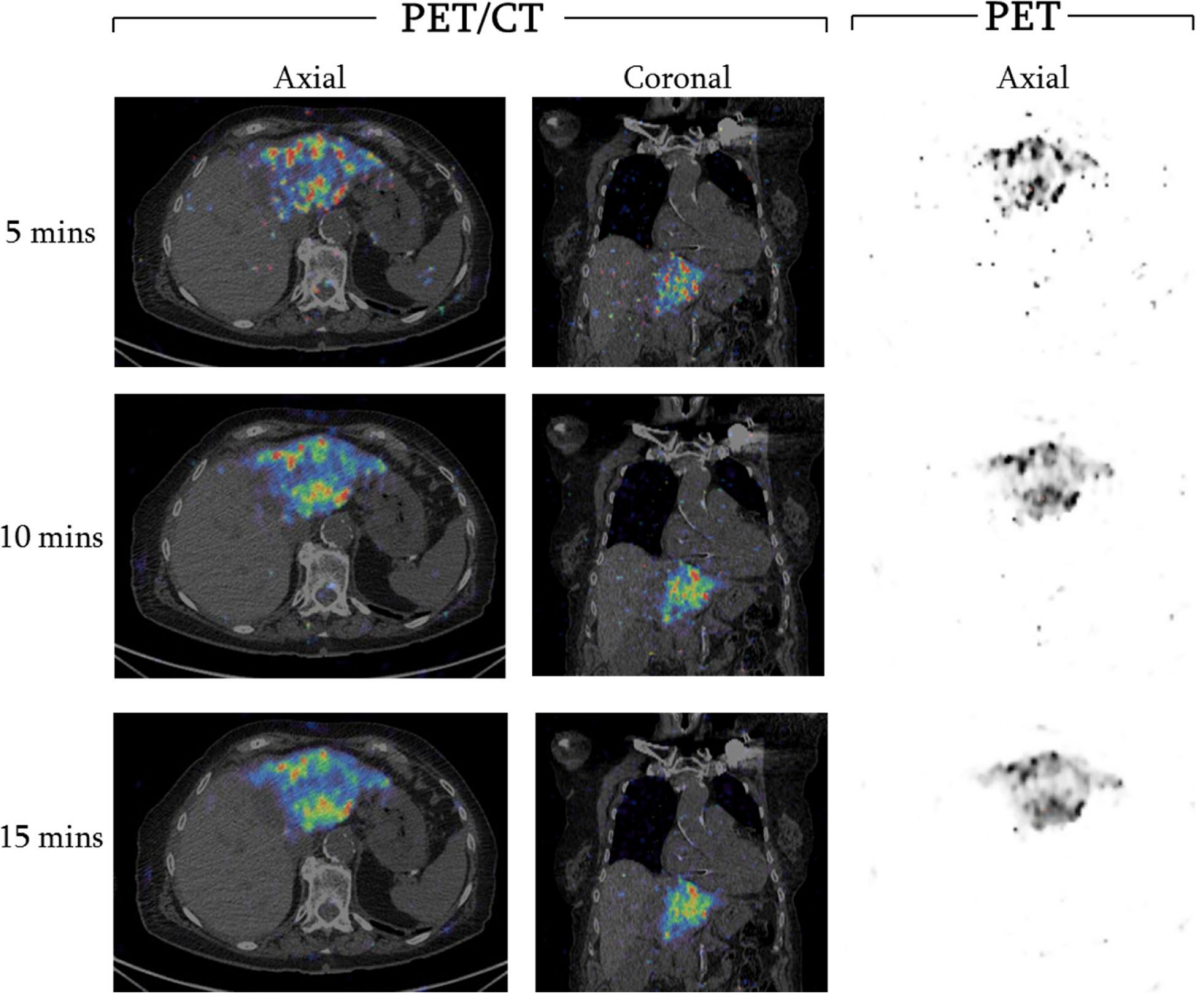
Images of varying acquisition lengths for a patient who had a PET/CT scan post-SIRT. Reconstructions have been performed using BPL with a beta value of 1000. Axial and coronal slices of the fused PET/CT images are displayed as well as axial slices of just the PET images. The same slices have been used for all acquisition lengths
